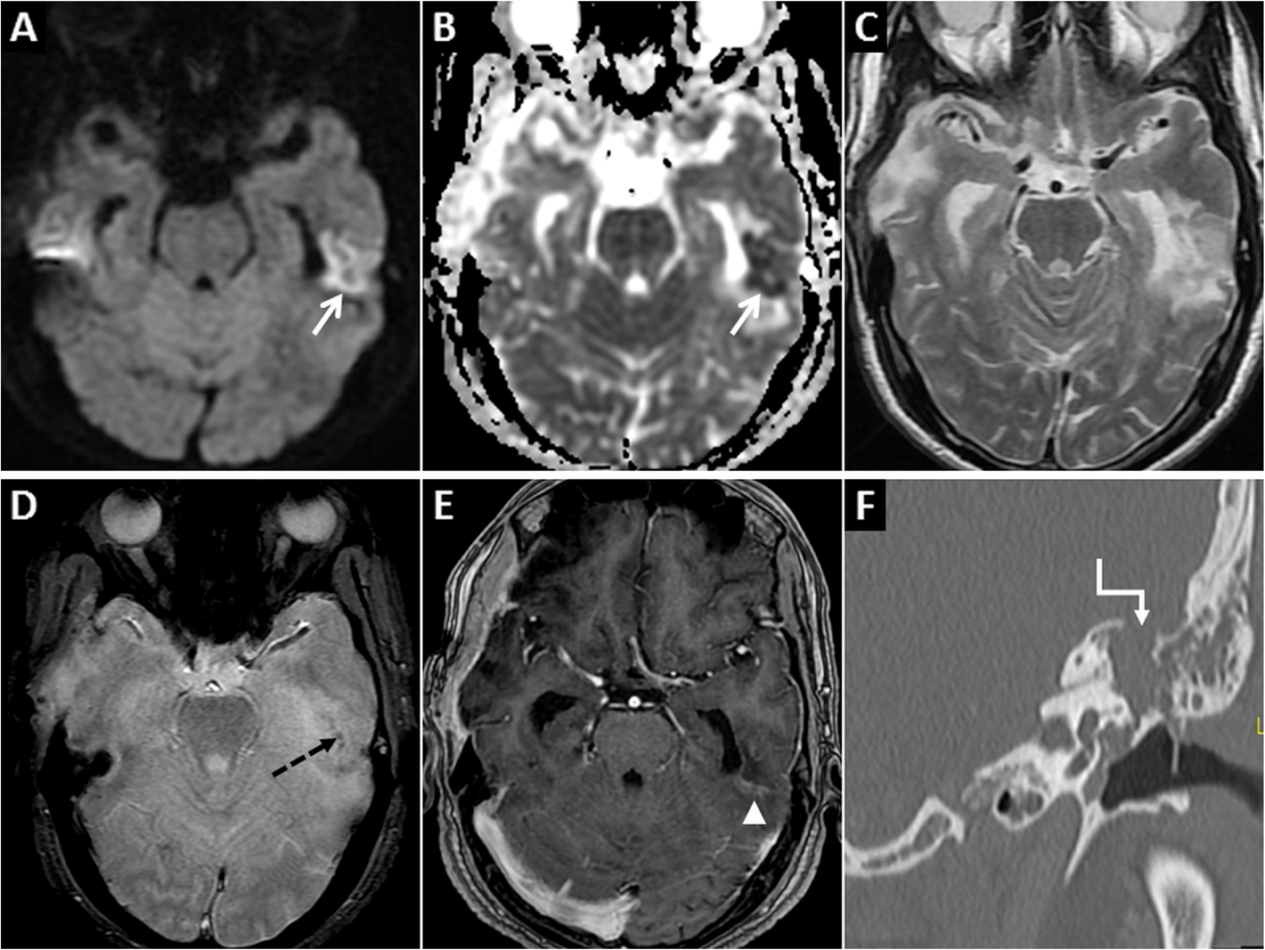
Pre and post-contrast MRI of the brain in a 70-year-old male patient who presented with altered mental status and discharging left ear. Localised GRD is seen in the posterior part of the left inferior temporal gyrus (arrows) on axial DWI (a) and ADC image (b) along with surrounding vasogenic oedema on axial T2W image (c). There are tiny haemorrhages (dashed arrow) in this lesion on axial GRE image (d) and subtle gyriform enhancement (arrowhead) on post-contrast axial T1W image (e). Coronal reconstruction of subsequent high-resolution CT of the left temporal bone (f) reveals extensive left otomastoiditis with a large bony defect in the left tegmen tympani (stepped arrow). Imaging findings are in keeping with complicated left otomastoiditis leading to cerebritis
